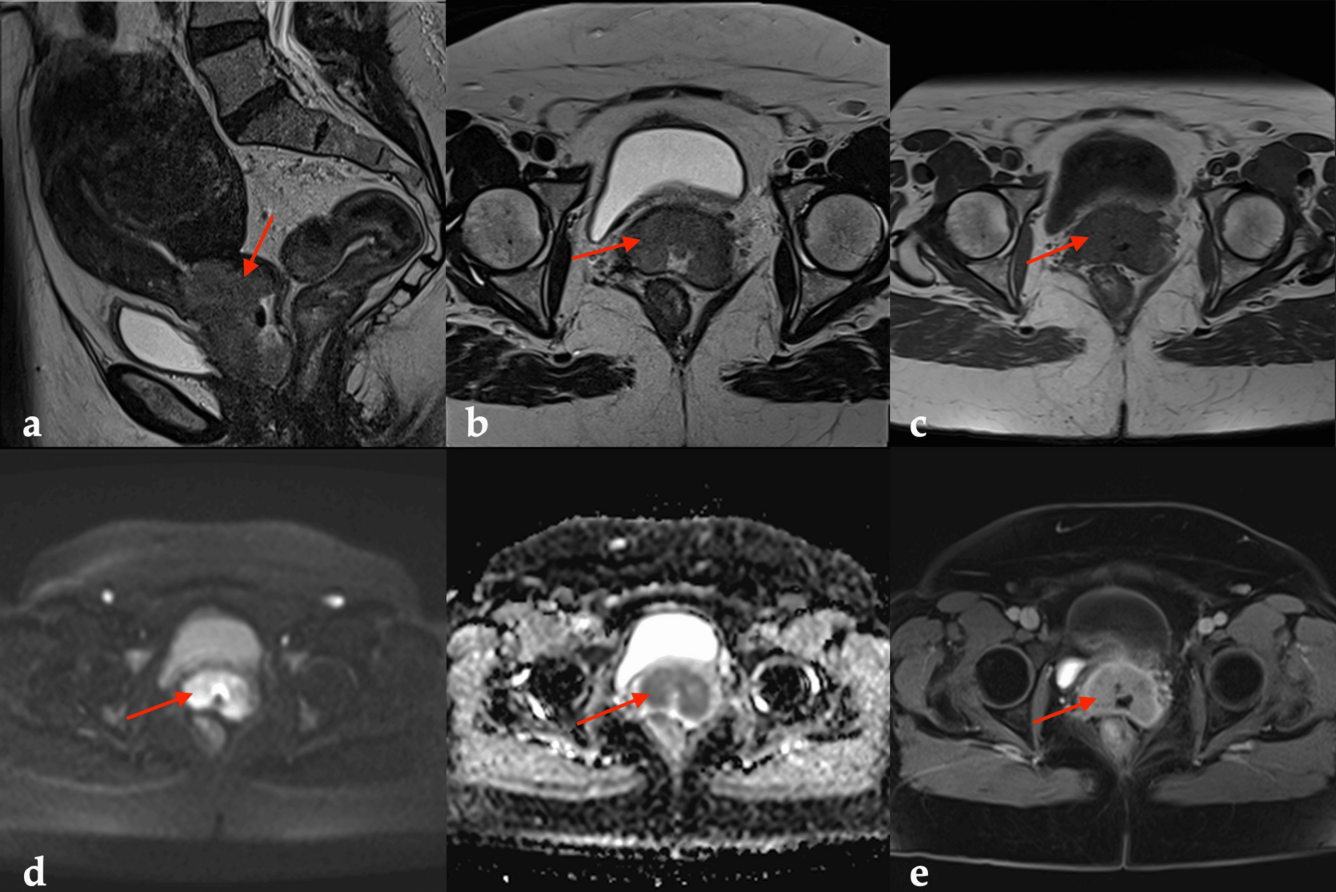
Fifty-three-year-old female patient complaining of post-menopausal bleeding. Pathology revealed endocervical adenocarcinoma. (a, b) Sagittal and axial T2WI showed hyper intense lesion; (c) Axial T1WI revealed isointense cervical lesion; (d) High signal on DWI and low ADC levels. ADC mean value was (0.78 × 10−3 mm^2^/second); (e) T1 FS postcontrast axial image with heterogeneous enhancement.  Red arrows point to the lesion. T2WI: T2 weighted imaging; DWI: diffusion-weighted imaging; ADC: apparent diffusion coefficient; T1 FS: T1 weighted fat-saturated
